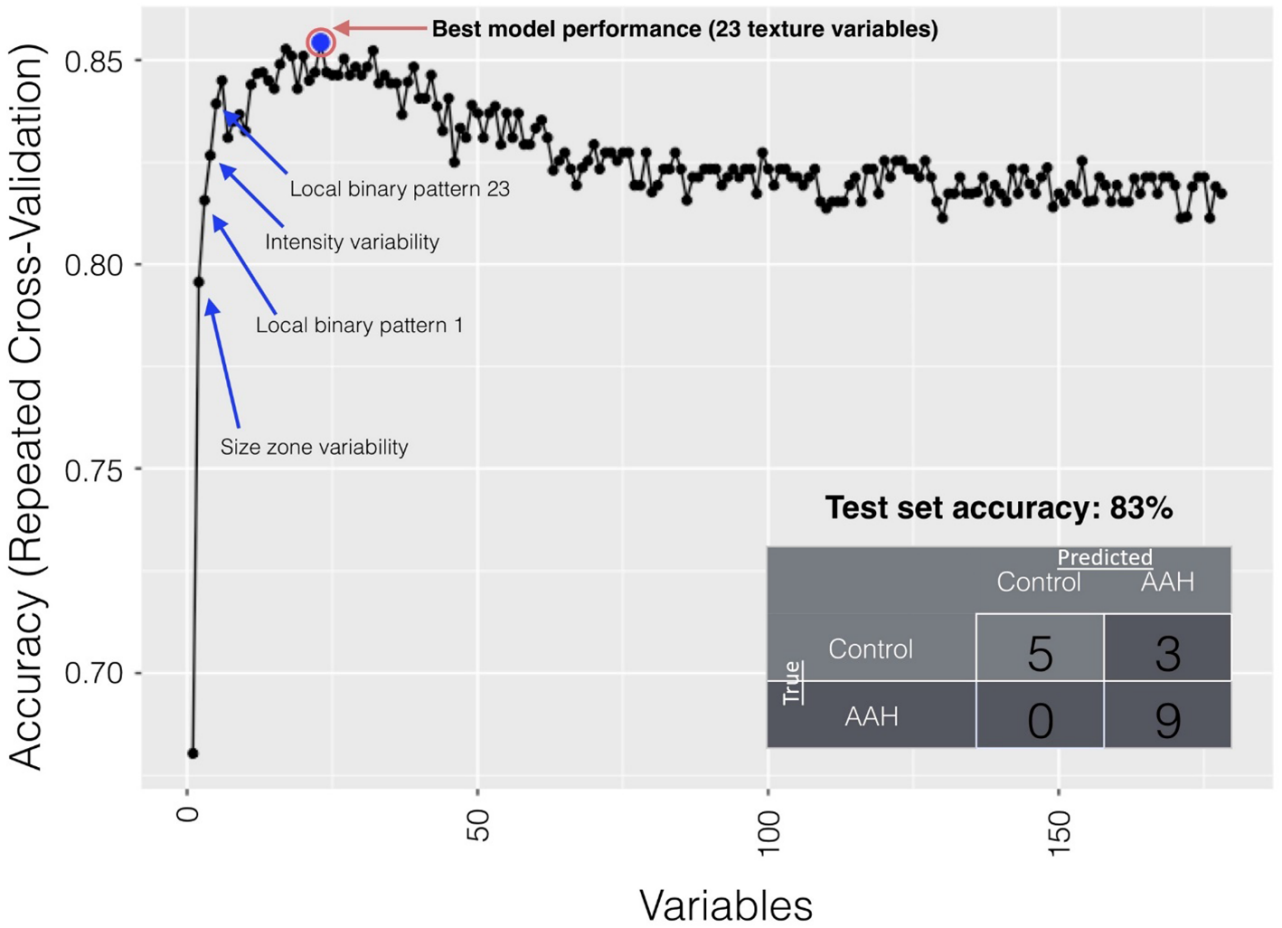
Results from the Recursive Feature Elimination using Random Forest (RFE-RF) algorithm, used to identify key CT liver texture features to differentiate between AAH and control patients. A total of 178 texture features, and a combination of 23 of these features were associated with the best model performance (85.4% accuracy in distinguishing AAH from control patients). These 23 texture features are listed in Table 3. The 23-feature model applied to the left-out test set is described in the bottom-right table and resulted in an accuracy of 82.4% (14 of 17 patients), sensitivity of 100%, specificity of 75%, NPV of 100%, and PPV of 62.75%.
